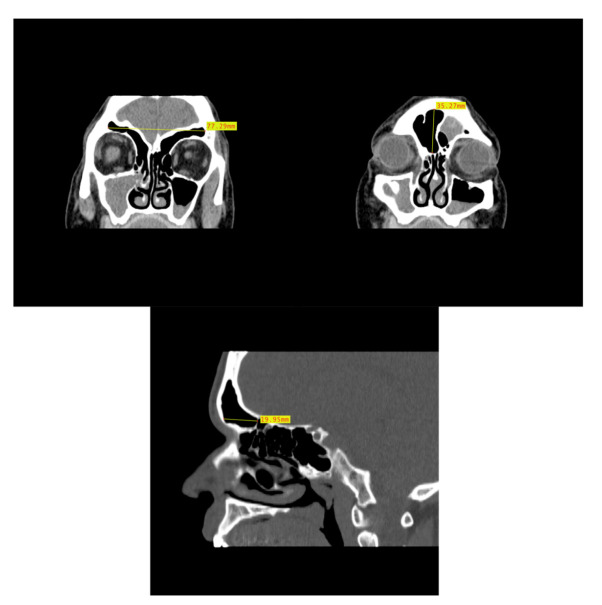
Example of CT image slice showing the maximum height (left) and width (right) measurement of the frontal sinus in the coronal plane and the frontal sinus maximum depth (bottom) in the sagittal plane from three study individuals (yellow lines and red boxes).
